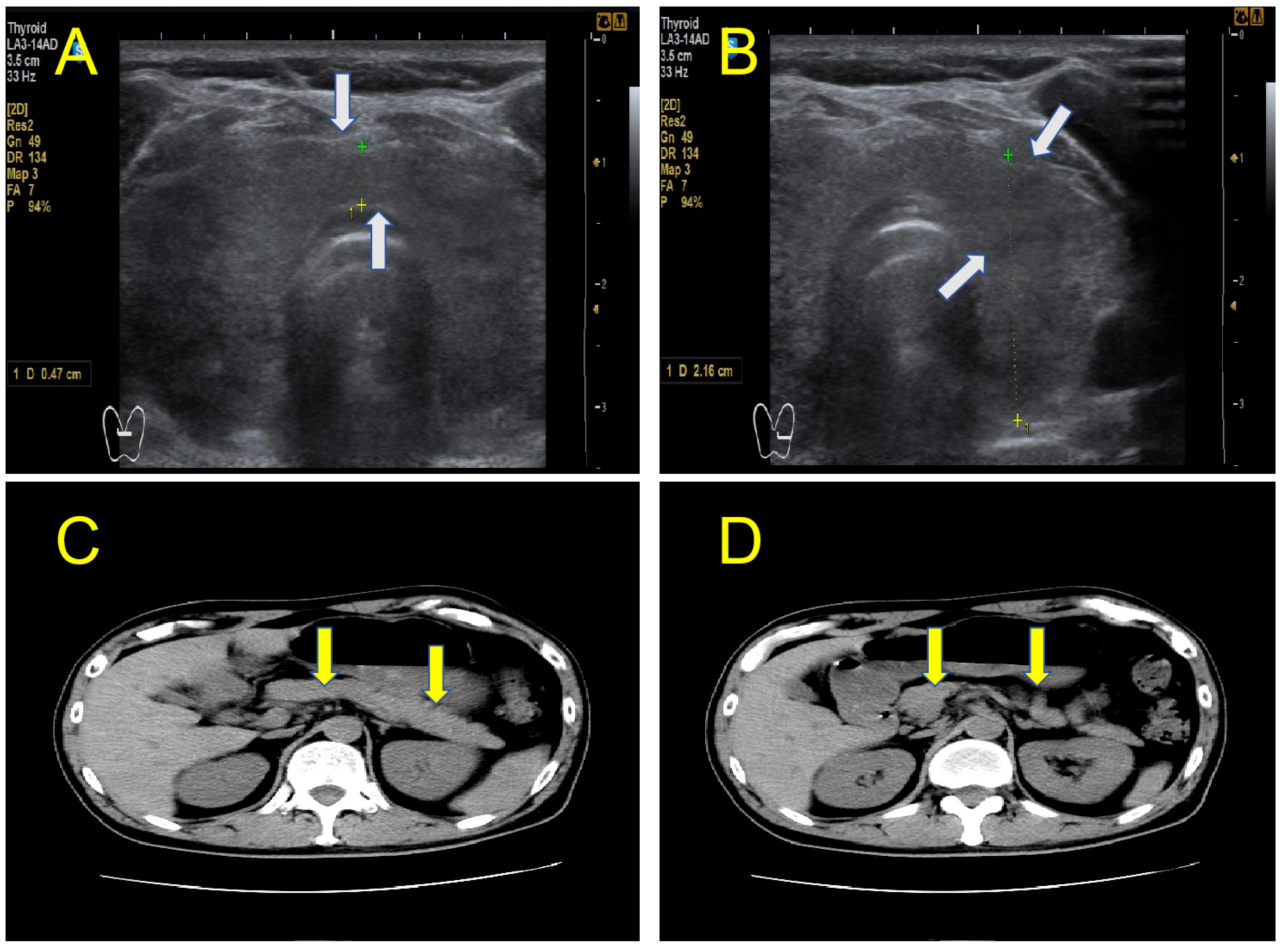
(A, B) Ultrasound showed local thyroid enlargement [gray arrow]. (C, D) The abdominal CT scan showed no morphological changes of the pancreas [yellow arrow].
